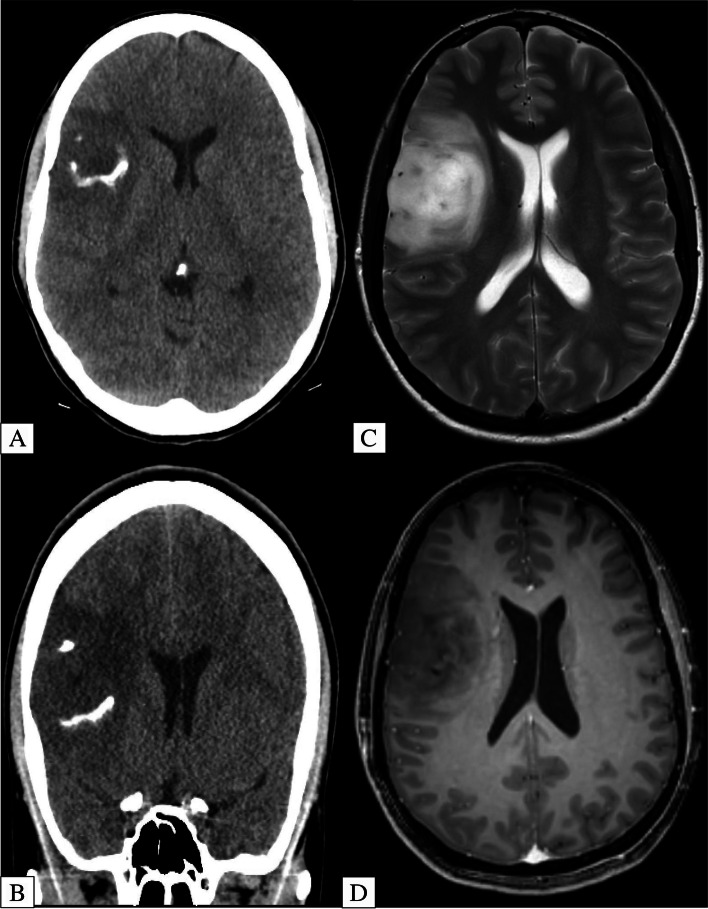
A-D 38-Year-Old Patient with Biopsy Proven Right Frontal Lobe Oligodendroglioma. Axial and coronal unenhanced CT (A/B) show a hypodense right frontal lobe lesion with gyriform/ribbon calcification. Axial T2-Weighted MRI (C) shows a corroborative T2 hyperintense lesion with no substantial enhancement on axial T1-Weighted Post Contrast MRI (D)
